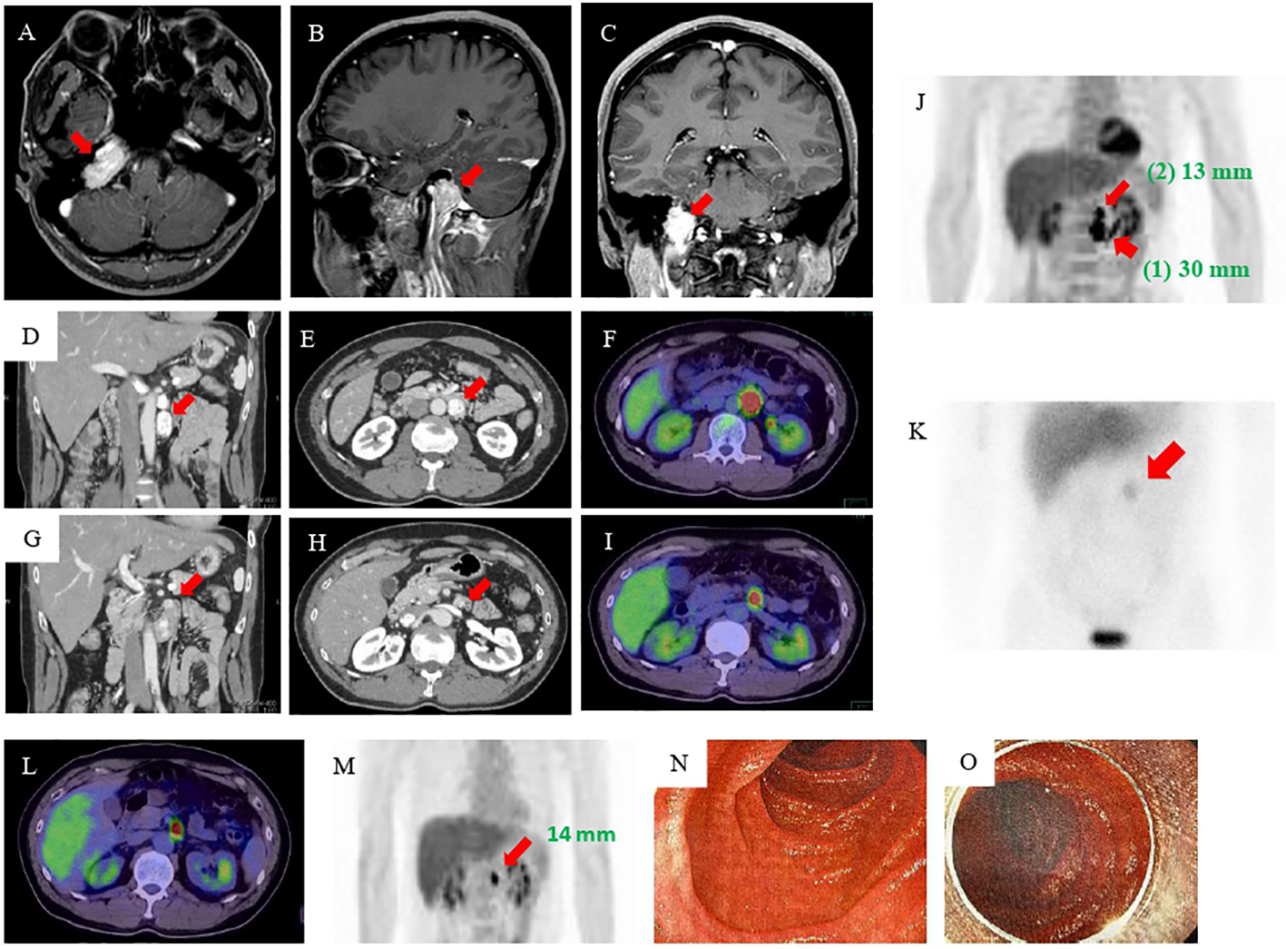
MRI, CT, 18F-FDG-PET, and MIBG imaging results. (A–C) MRI of the head revealed an approximately 3-cm mass at the right transvenous foramen. (D, E) Enhanced abdominal CT revealed two tumors in the retroperitoneum: a nodal tumor measuring 30 mm (arrow) on the anterior left renal vein and (G, H) a tumor measuring 13 mm (arrow) near the ligament of Treitz. (F, I, J) 18F-FDG-PET showed accumulation in both tumors [(1) the tumor measuring 30 mm (wide arrow) and (2) the tumor measuring 13 mm (narrow arrow)]. (K) MIBG scintigraphy showed accumulation in an area consistent with a mass (arrow). (L, M) Esophagogastroduodenoscopy and enteroscopy did not identify the smaller tumor. (N, O) MRI, magnetic resonance imaging; CT, computed tomography; FDG-PET, fluorodeoxyglucose-positron emission tomography; MIBG, 123I-metaiodobenzylguanidine.
